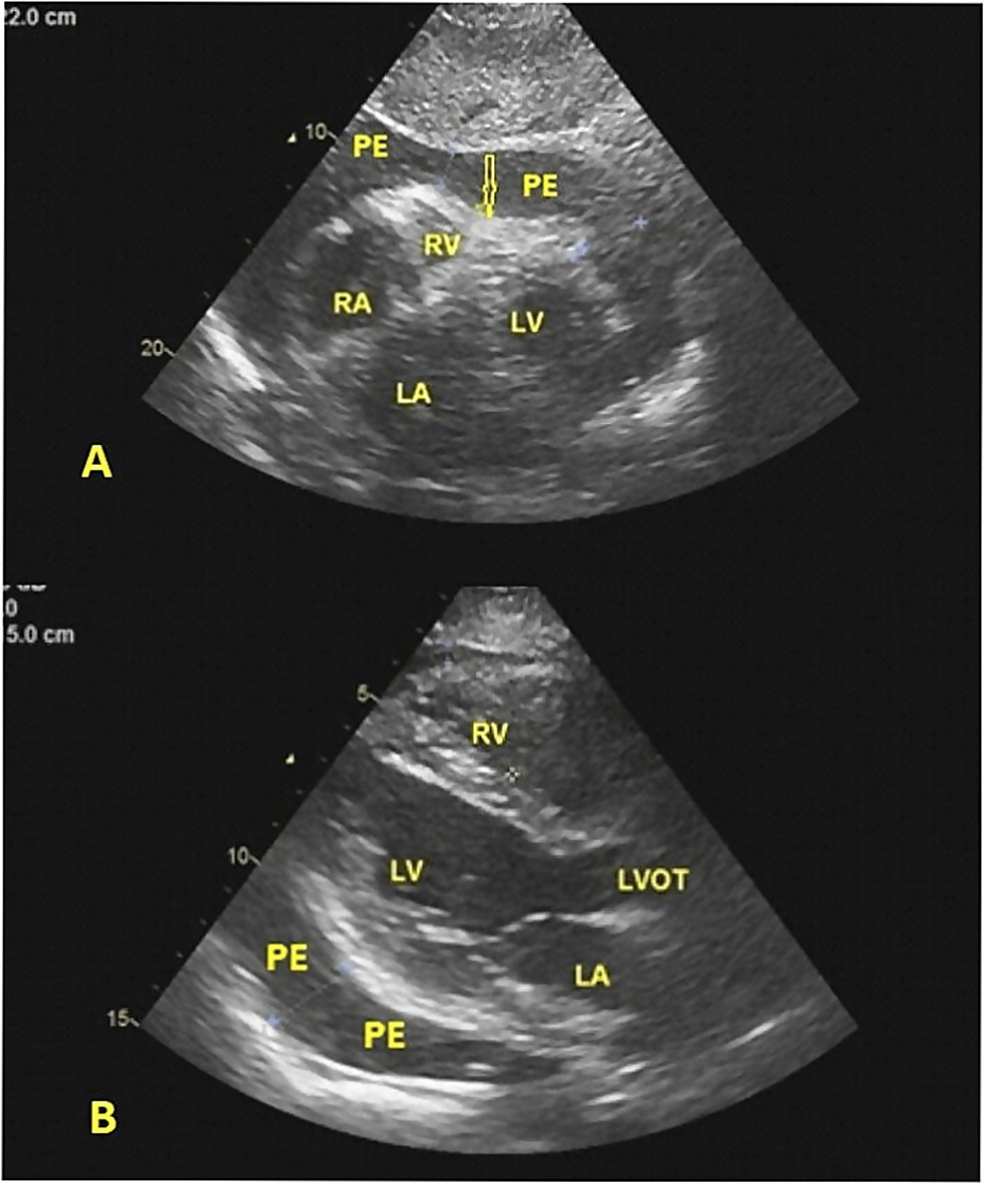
(A) Transthoracic 2-dimensional echocardiogram (subcostal view) showing a large pericardial effusion (PE) and diastolic collapse of the right ventricular free wall (arrow). (B) Transthoracic 2-dimensional echocardiogram (parasternal long axis view) showing pericardial effusion (PE) behind the left ventricular (LV) posterior wall.
